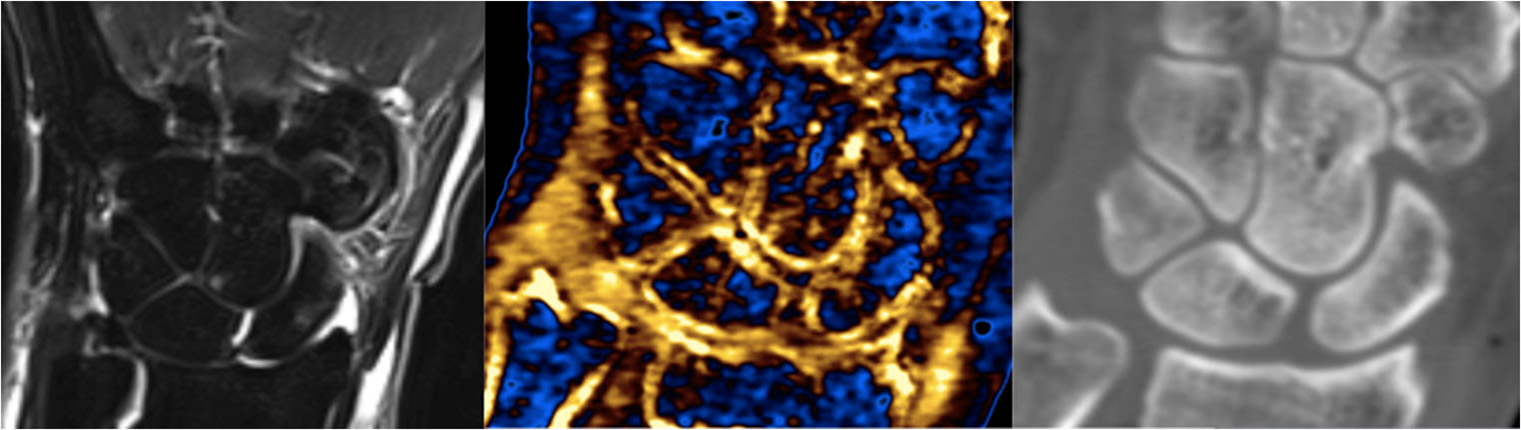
Mild acute scaphoid oedema. The STIR sequence of the MRI (left) showed a small peripheral area of oedema in the region of the waist of the scaphoid. This was difficult to detect with confidence on the DECT with calcium/water filter (middle) due to the ‘blooming’ artefact from the bony cortex. No fracture seen on the CT component (right)
